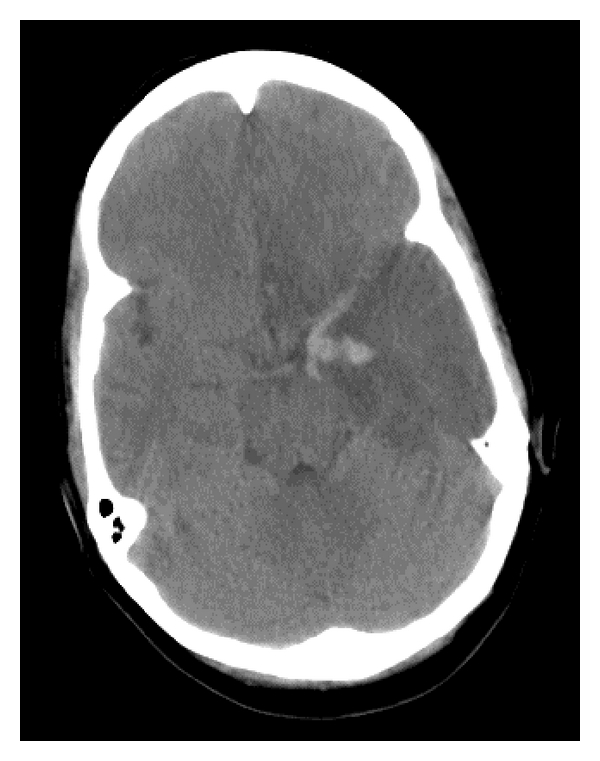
CT head: left temporal lobe hypodensity with a discrete area of hemorrhage.
